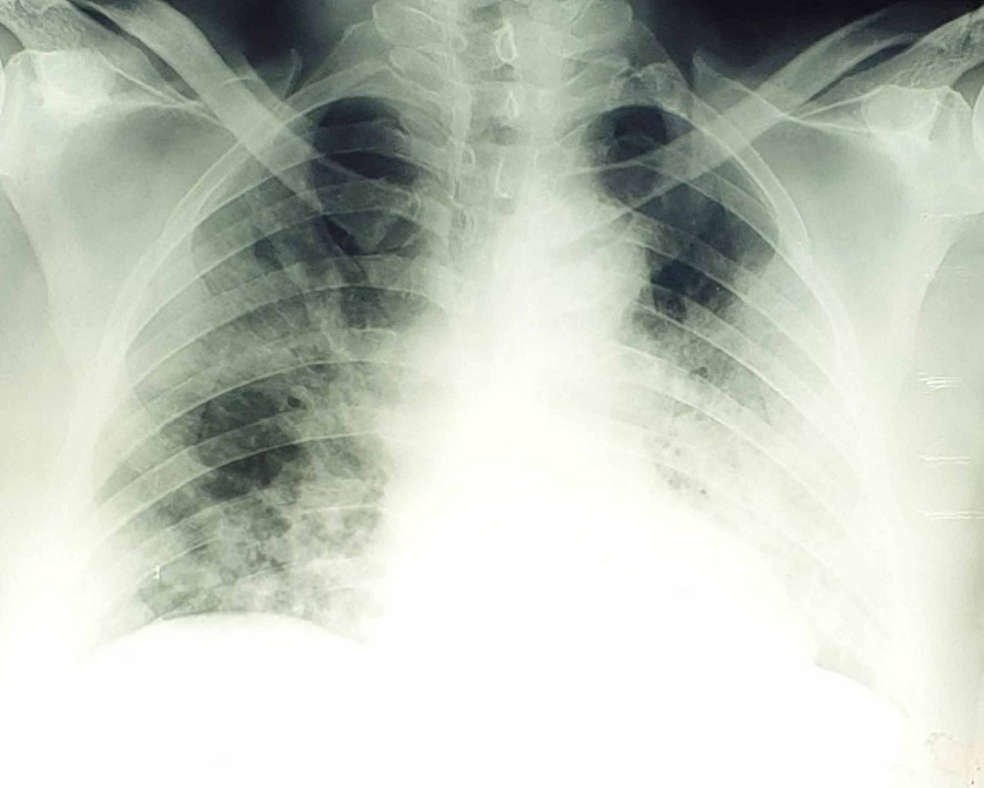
Chest radiograph of the patient with COVID-19 at diagnosis.
